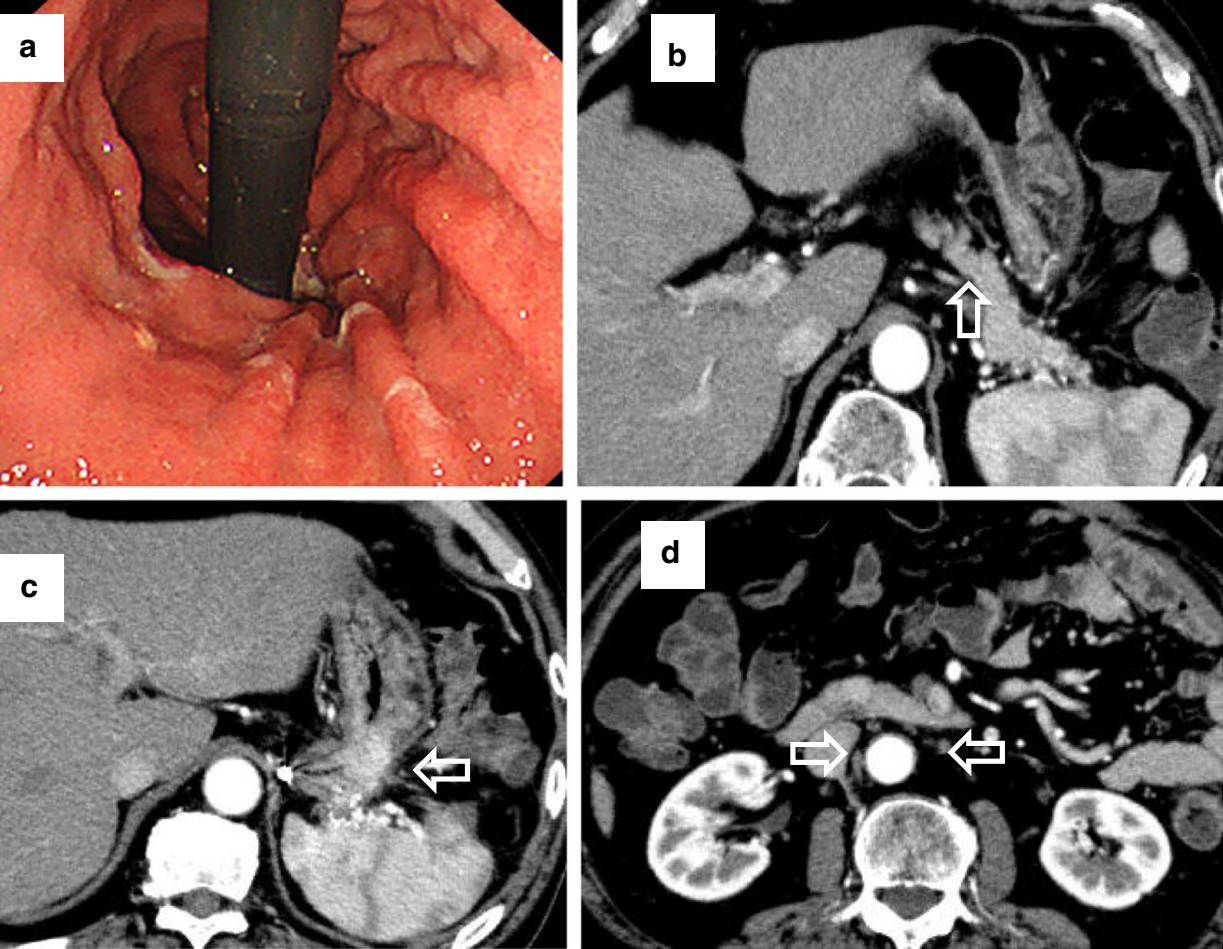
Endoscopic and computed tomography (CT) images after nivolumab therapy. a Endoscopic image showing that the primary lesion had decreased markedly in size. b CT image showing decreased invasion of the pancreas (white arrows). c CT image showing decreased invasion of the spleen, and smaller lesser curvature lymph nodes (white arrows). d CT image showing that the para-aortic lymph nodes are inconspicuous (white arrows)
